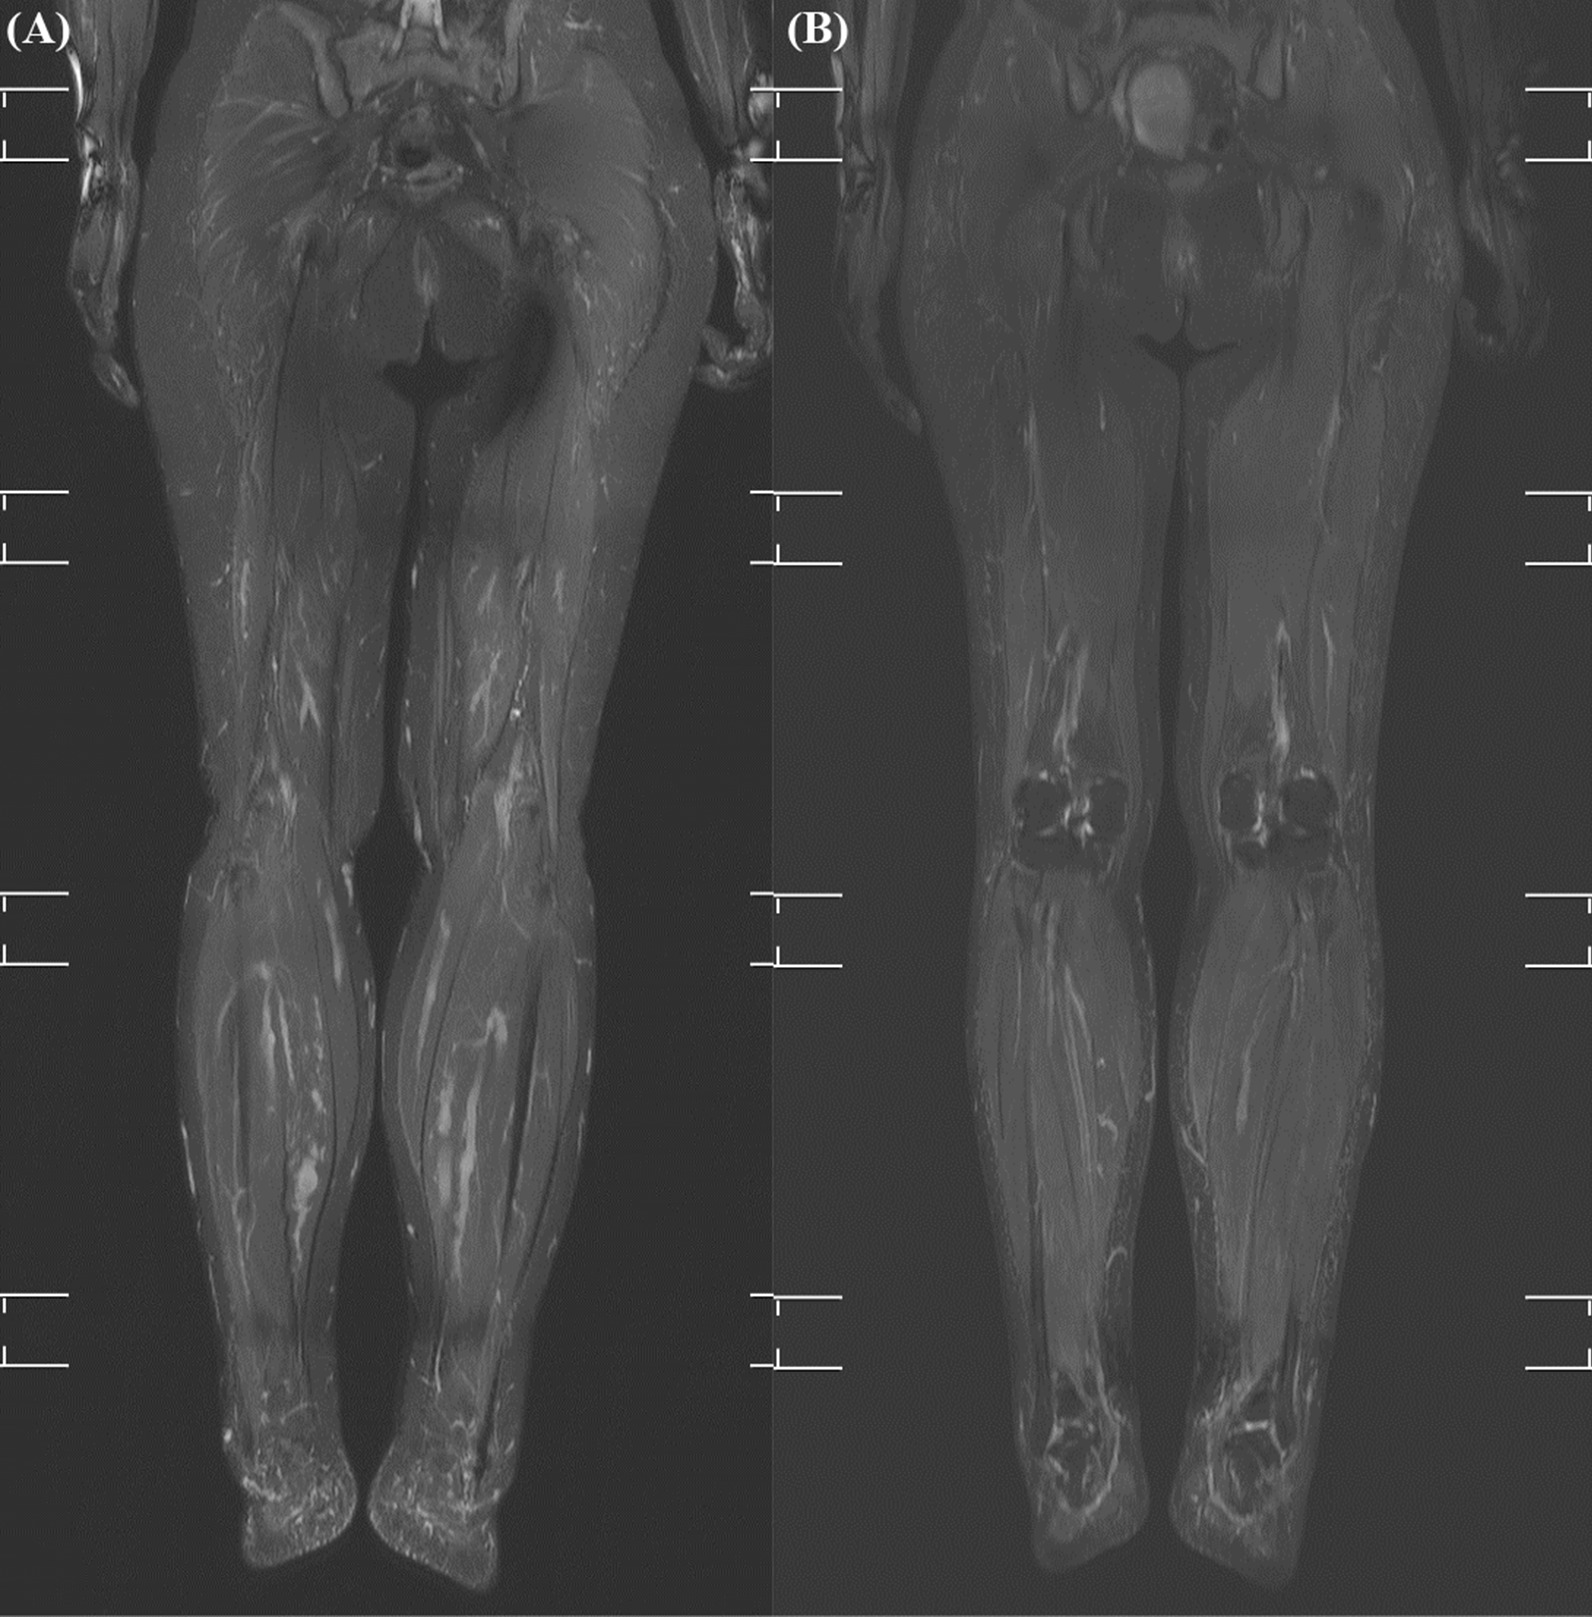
Change in the MRI findings of patient 3 after the administration of alpelisib. A The MRI image of both lower legs before treatment B The MRI image of both legs after treatment. The extent of fine stranding of the subcutaneous layer of the bilateral distal lower legs decreased. Minimal improvement of the tortuous and dilated deep and superficial venous structure in the lower extremities was observed
